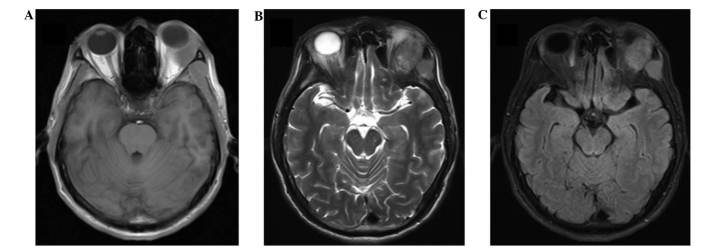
Magnetic resonance imaging of the patient. The isointensity of the lesion is visible on (A) T1-weighted, (B) T2-weighted and (C) fluid-attenuated inversion recovery images.
